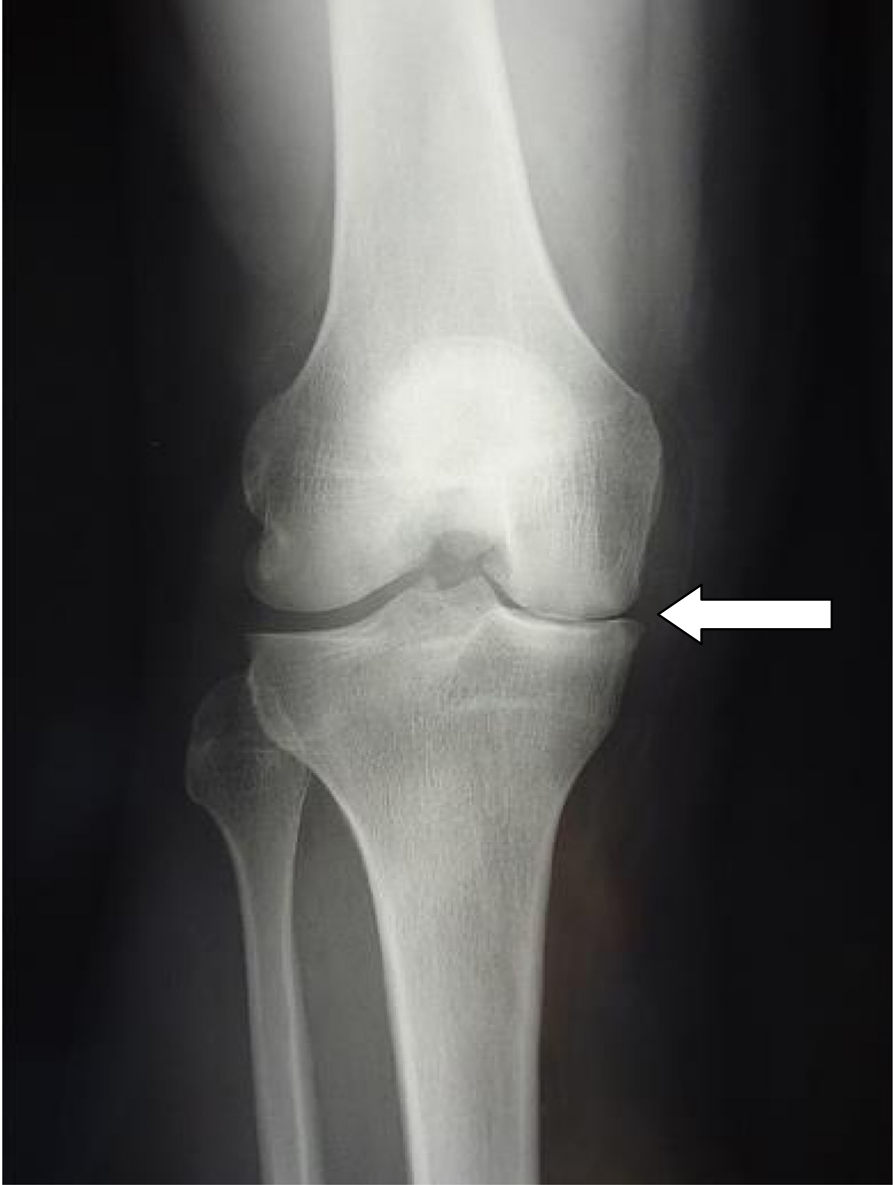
Postoperative radiography at 3 month follow-up. Anteriorposterior view, lateral view is not available. Complete medial femorotibial thinning (arrow)
